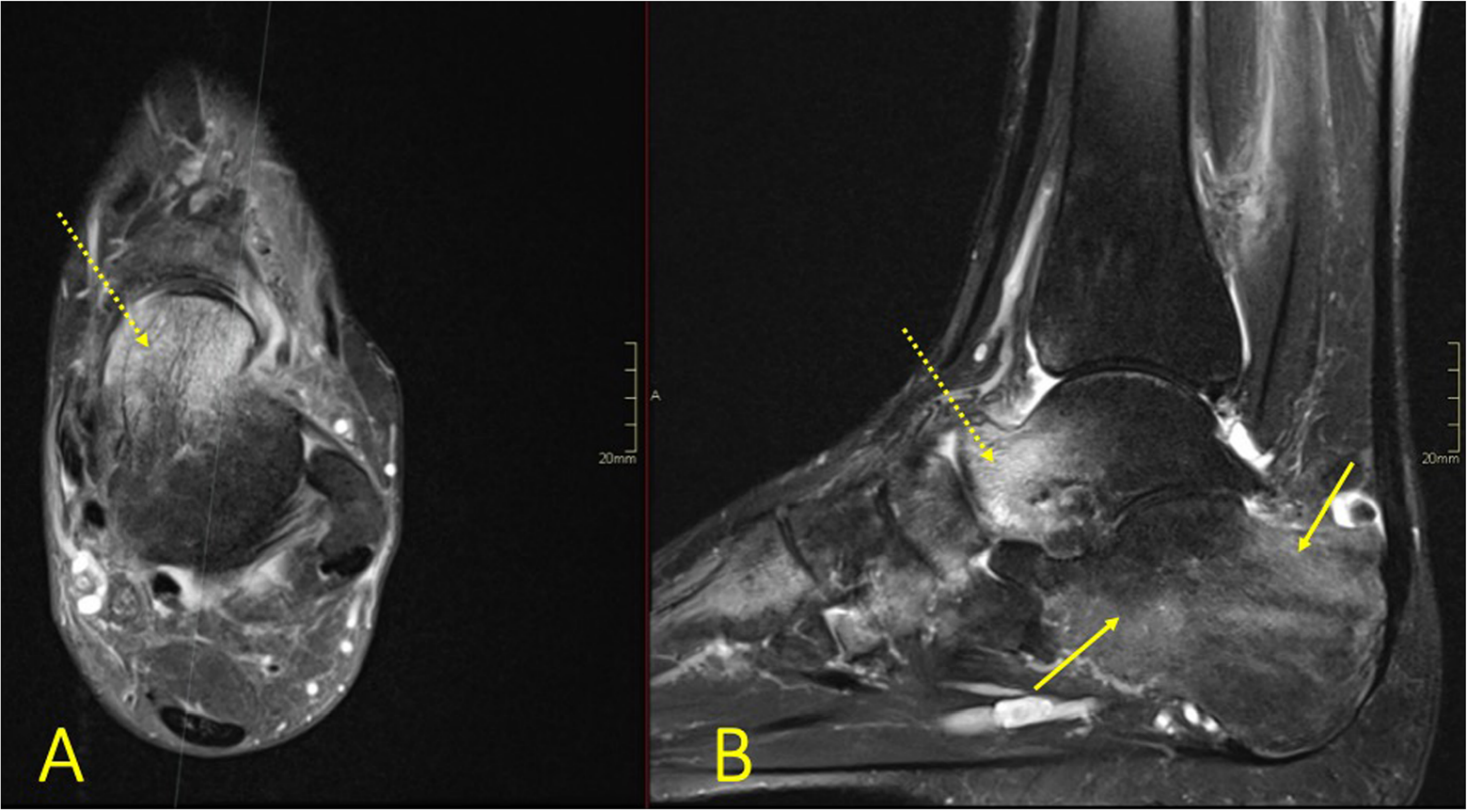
A 70-year-old female with diabetes and Charcot foot. MRI showed extensive bone marrow edema (dashed arrows) in all bones of the foot suggesting active Charcot changes, most prominent at the level of the Lisfranc joint, where dorsal dislocation of os cuneiforme intermedium is visible (arrow)
